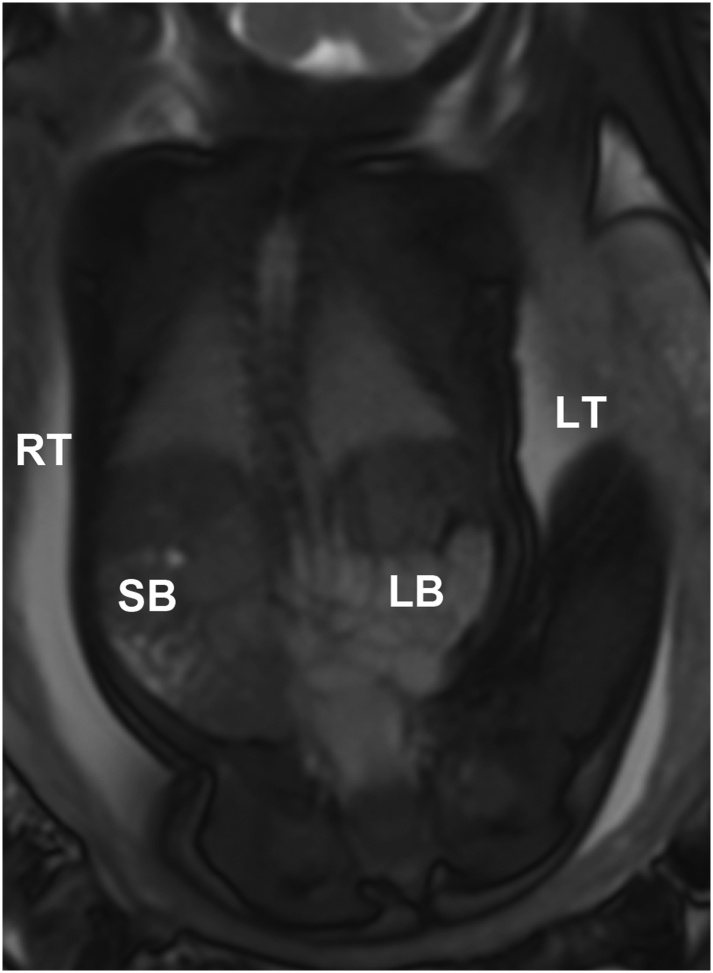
MRI imaging of 39 week fetus showing the bowel non-rotation in a coronal plane. SB = small bowel. LB = large bowel. LT = left side of the fetus. RT = right side of the fetus.
